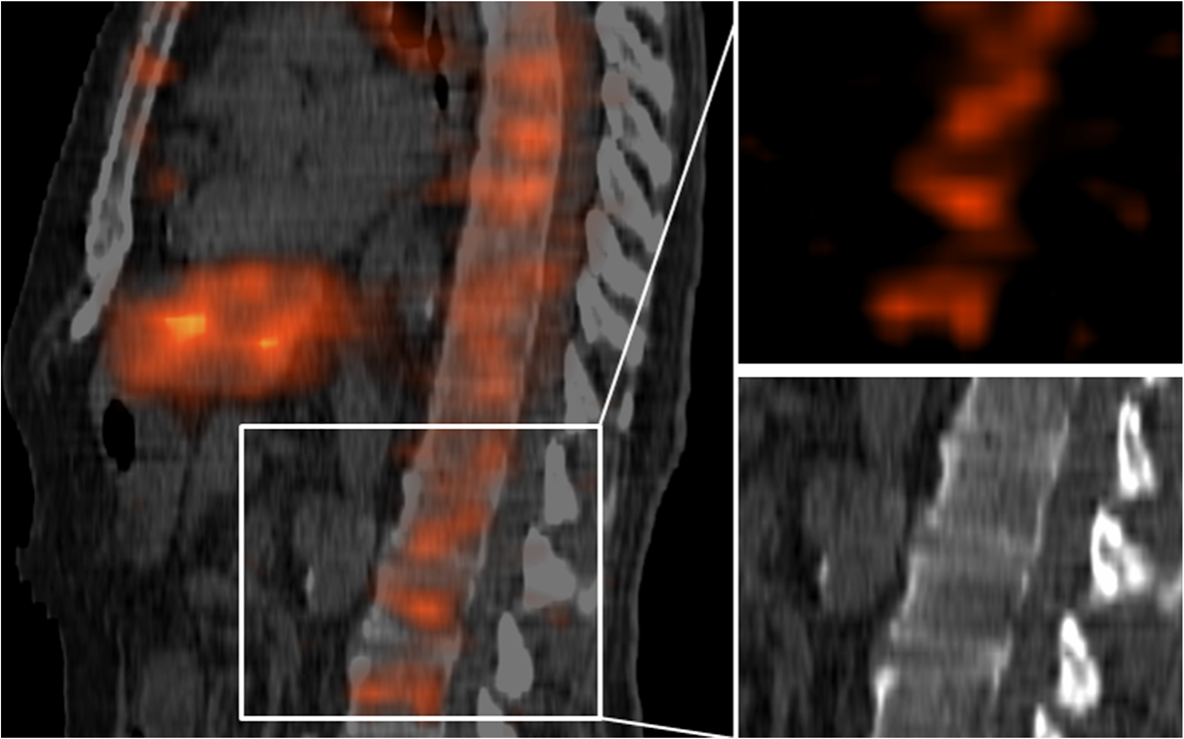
Accumulation of activity in the RBM. (Left) The sagittal cross section of low-dose CT and the SPECT image made at 72 h after administration of the ^177^Lu-peptide of patient 20. (Right) The SPECT and low-dose CT images of vertebrae in which activity accumulated.
